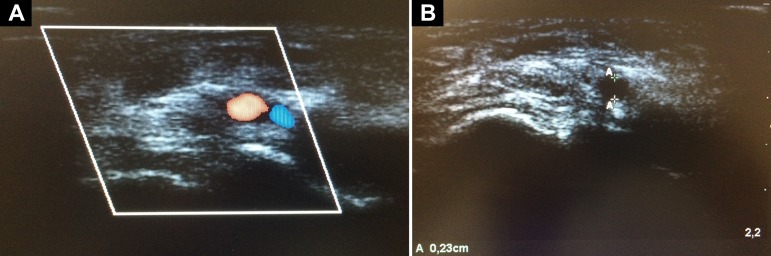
Ultrasonographic evaluation of the radial artery. (A) Cross-sectional view of the radial artery with color Doppler. (B) Measurement of the diameter of the dorsal radial artery.
